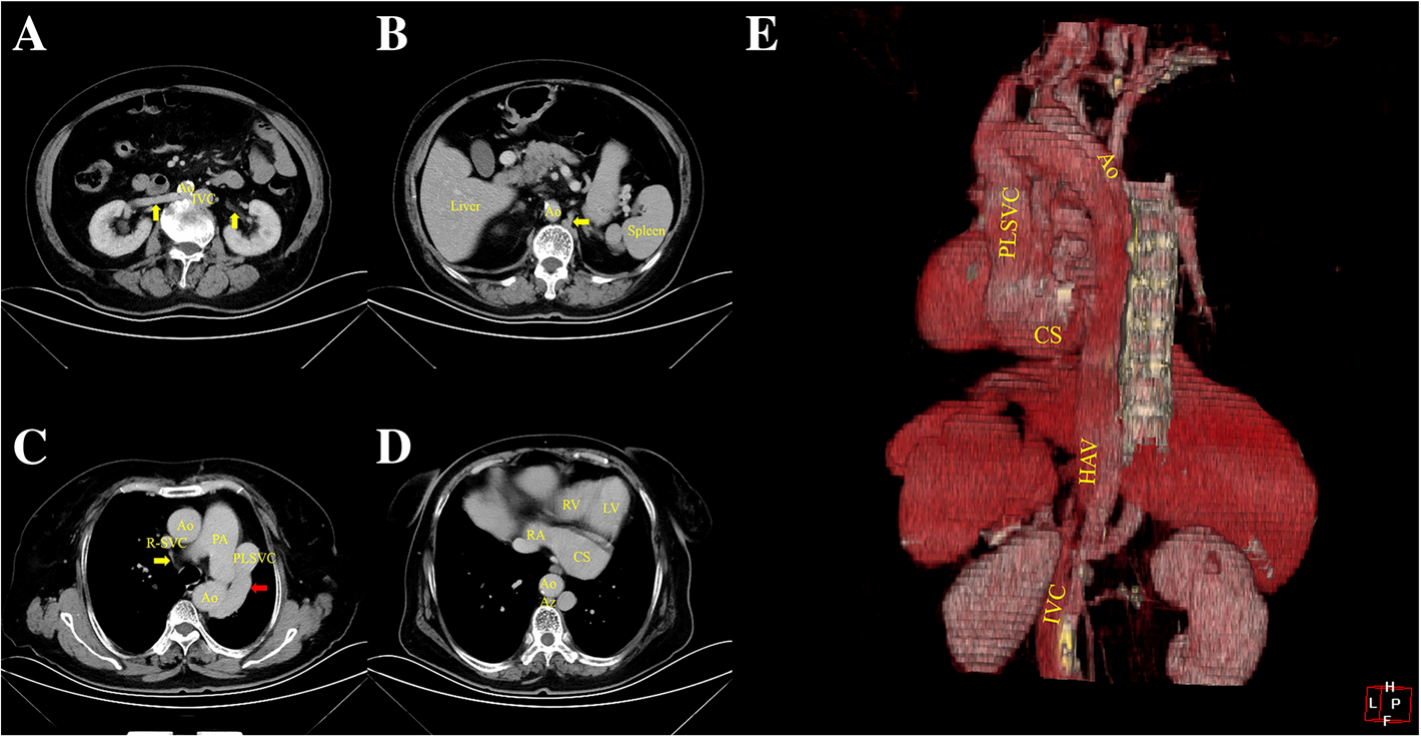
Thoracic and abdominal enhanced computed tomography demonstrating (a) A left IVC posterolateral to the abdominal aorta, converged by two renal veins (yellow arrow). b The left IVC gradually becomes thin, and continues with an enlarged hemiazygos vein (yellow arrow). Visceral position is normal. c The tiny azygos vein can be seen anterior to the vertebra, then crosses over the aorta and drains into the R-SVC (yellow arrow). The hemiazygos vein continues as the left superior intercostal vein via the accessory hemiazygos vein. The left superior intercostal vein eventually drained into the PLSVC at the level of the pulmonary artery bifurcation (red arrow). d In the left atrioventricular groove, a huge CS connected with the RA. e Reconstructive 3D imaging gives a better view of this anomaly. Ao: aorta; Az: azygos vein; CS: coronary sinus; HAV: hemiazygos vein; IVC: inferior vena cava; LV: left ventricle; PA: pulmonary artery; PLSVC: persistent left superior vena cava; RA: right atrium; R-SVC: right superior vena cava; RV: right ventricle
